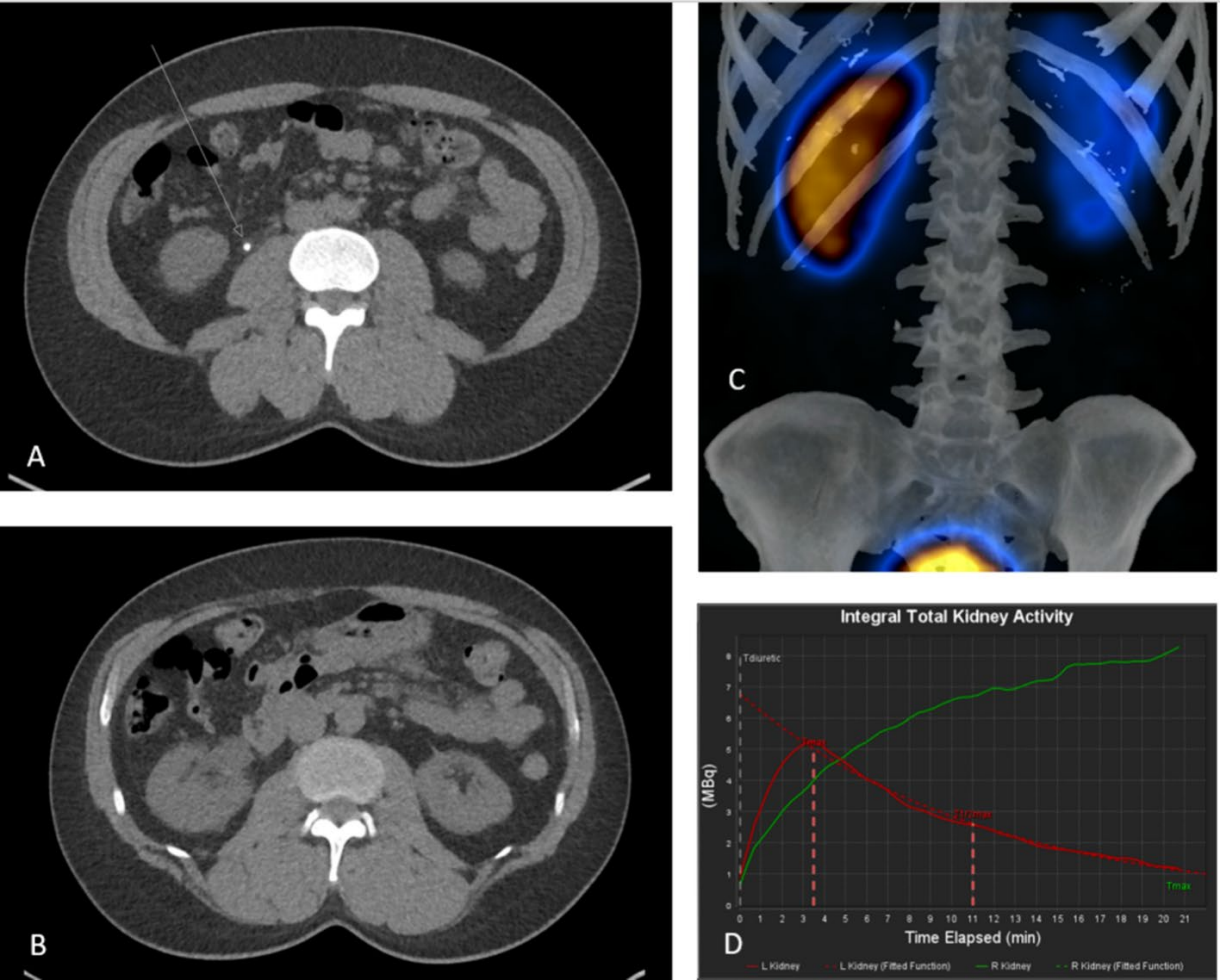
Images from case 18 in Table 2. The patient presented to the Emergency Department with renal colic symptoms, right flank pain and haematuria. A Axial UECT shows a stone in the proximal right ureter a maximum diameter of 3 mm (arrow). B Axial UECT at kidney level shows hydronephrosis of the right kidney. C 3D SPECT/CT reconstruction showing high tracer concentration in the right kidney while the left kidney has an adequate tracer elimination. D Renogram curves showing a falling curve from the left kidney (red curve) and an obstructive curve from the right kidney (red curve)
